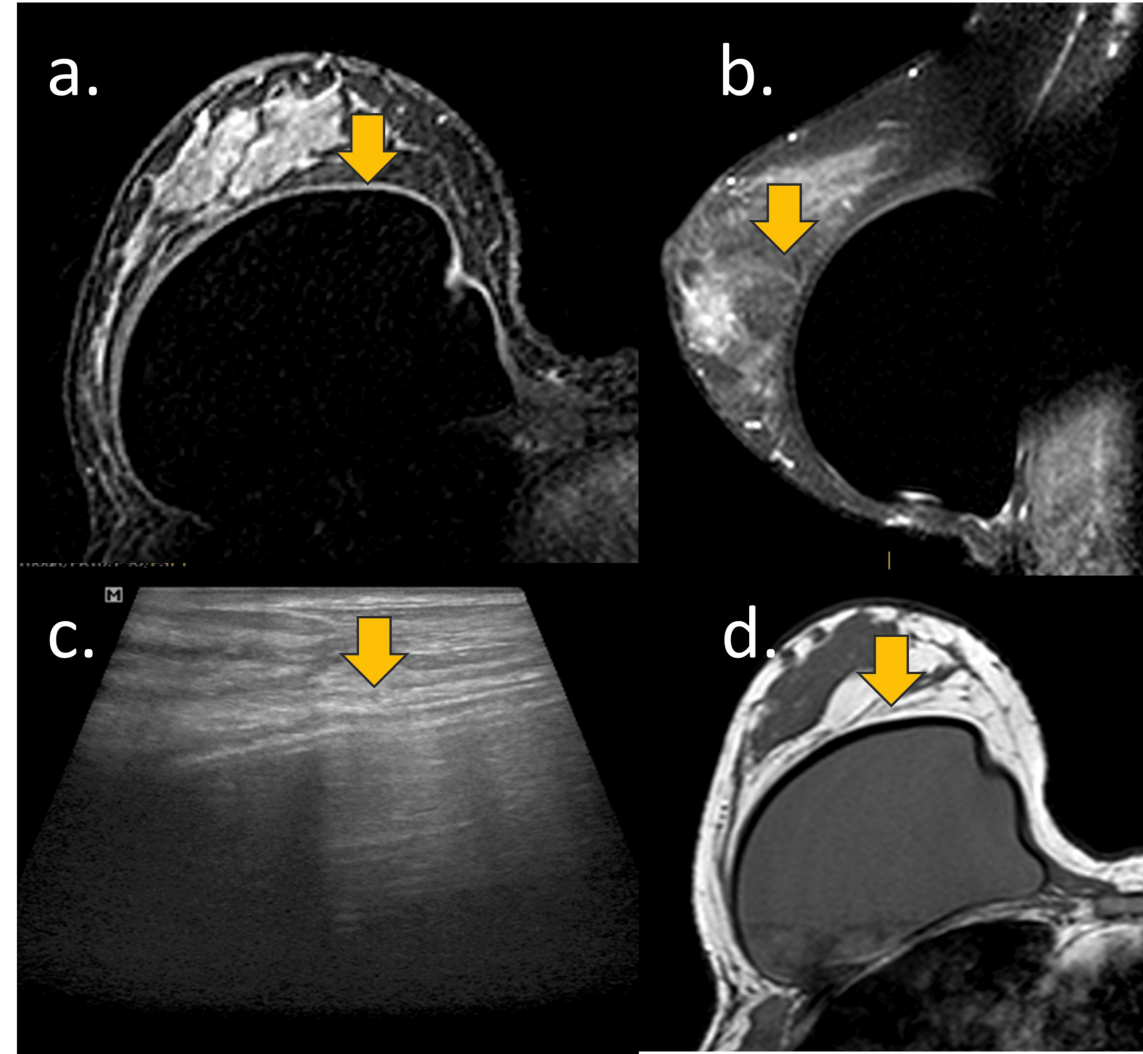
Expected acelullar dermal matrix (ADM) with silicone implants A 52-year-old woman with a retropectoral implant and ADM for five years. (a) Axial T1-weighted sequence, (b) sagittal STIR-weighted sequence, (c) breast USG, and (d) axial proton density-weighted sequence. (a, b, c, and d) The ADM is expected to integrate perfectly with the silicone implant and the fibrous capsule, remaining almost invisible to imaging methods, as shown by the yellow arrow. In these cases, the mammogram cannot be viewed. On MRI, pericapsular laminar tissue with marked hyposignal can be observed in all sequences. By studying ultrasound, the ADM can determine the attenuation of the sound beam.
